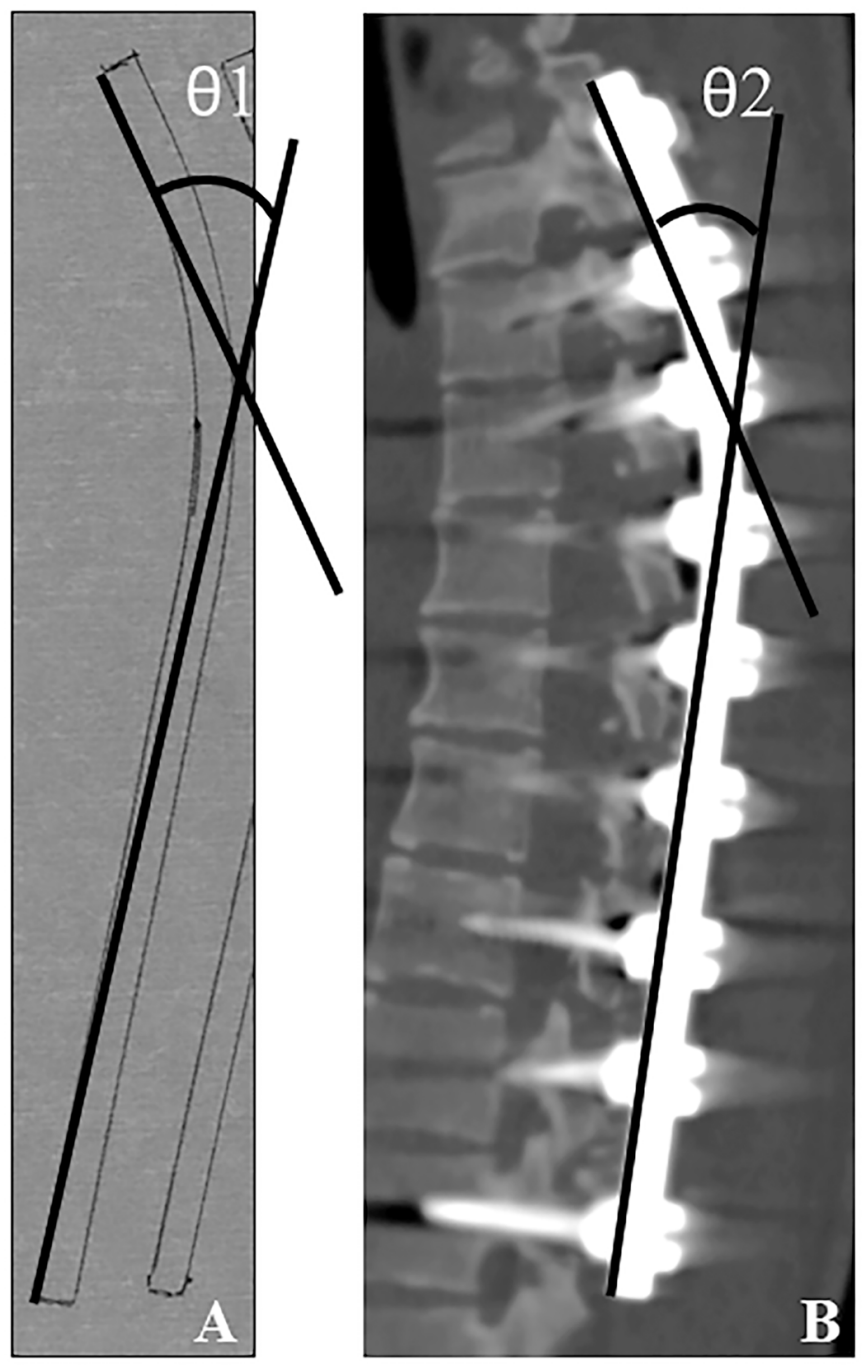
Rod angle before and after implantation. (A) Prior to implantation, the surgeon traced the rod shapes on paper. The angle between the proximal and distal tangential line was measured (θ1). (B) Postoperative implant rod geometry (θ2) was obtained after the surgical operation using computed tomography.
